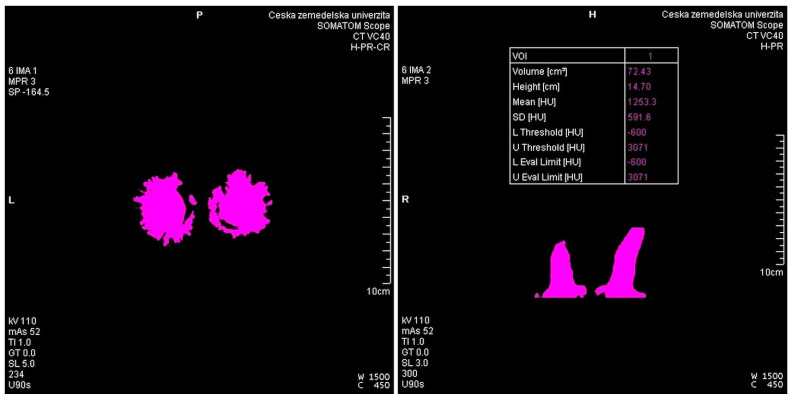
Measuring antler volume with a CT scanner.
